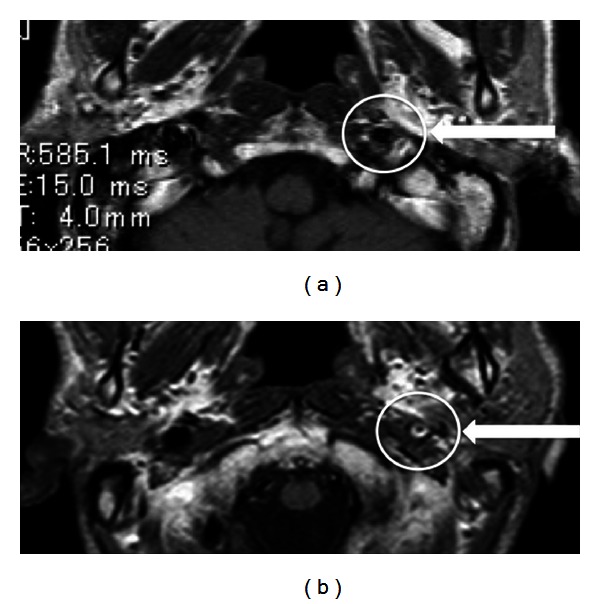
MR imaging (T2). (a) A flow void at the left carotid artery was observed immediately after radiotherapy. (b) No flow void was seen 1.5 years after radiotherapy, suggesting occlusion of the carotid artery.
